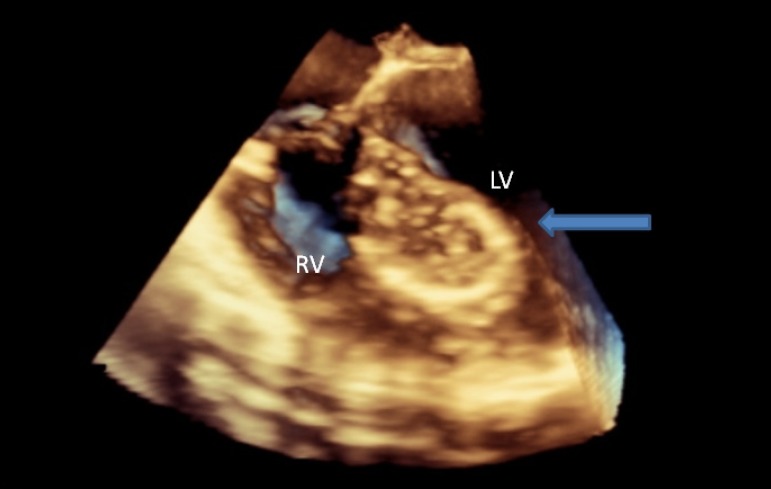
Echocardiographic view of the hydatid cyst. LV=left ventricle; RV=right
